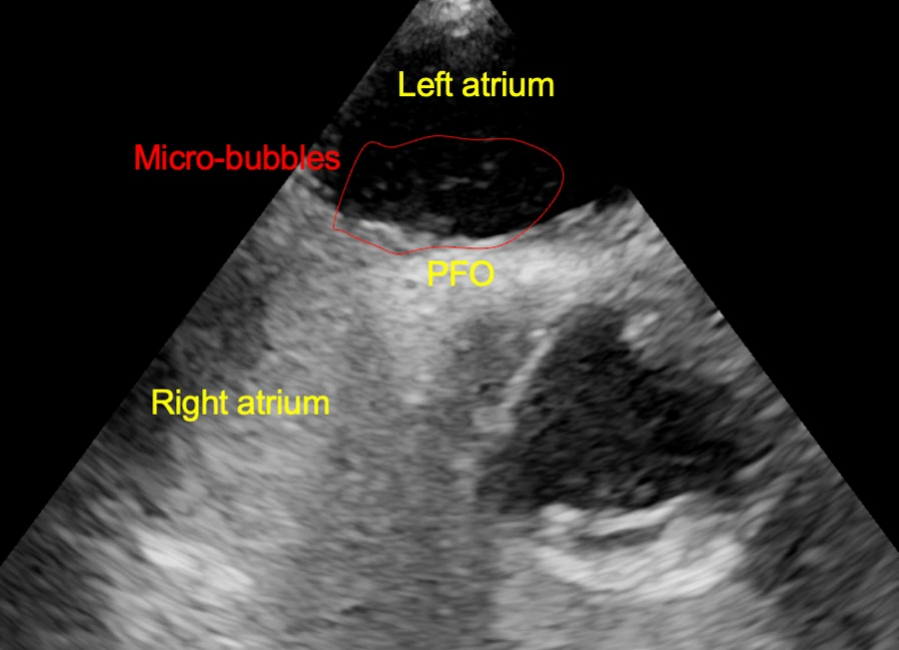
Postoperative transesophageal ultrasound examination affording two- and four-chamber long-axis views of the heart. A small atrial septal defect was seen (ovale) by micro bubble test
